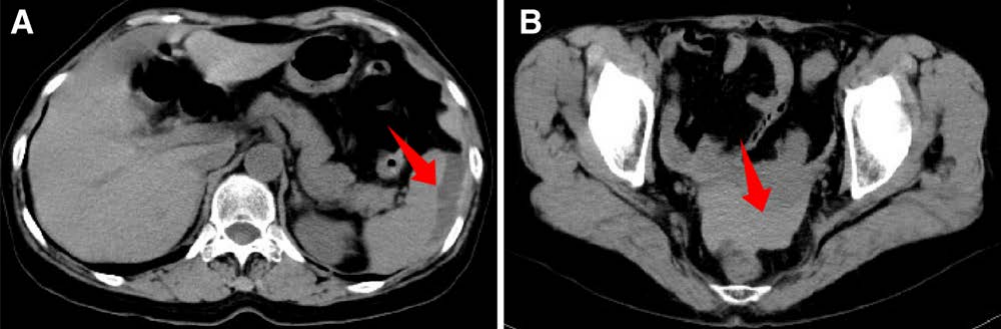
Postoperative day 3 CT images: (A) Abdominal CT shows a continuous arc-shaped low-density area under the splenic capsule (61 × 14 mm), representing a subcapsular hematoma with no signs of active bleeding (blush sign). (B) A high-density area in the rectouterine pouch (68 × 23 mm) indicates a pelvic hematoma. CT = computed tomography.
